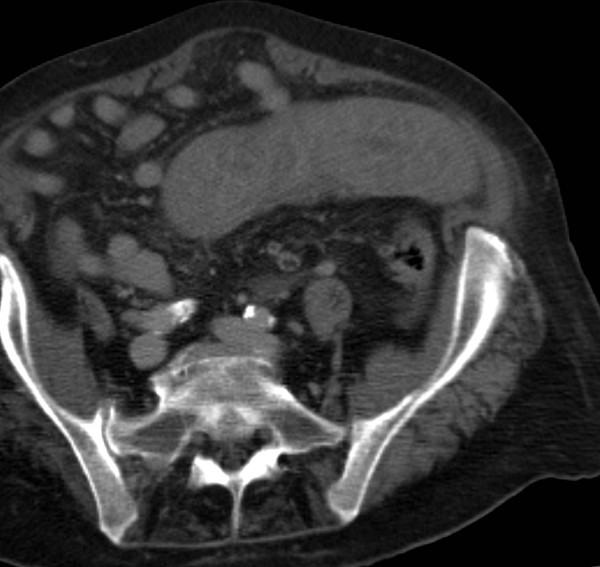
Axial CT scan. The grossly thickened loop of jejunum on the left side of the abdomen is typical of intramural haematoma.
